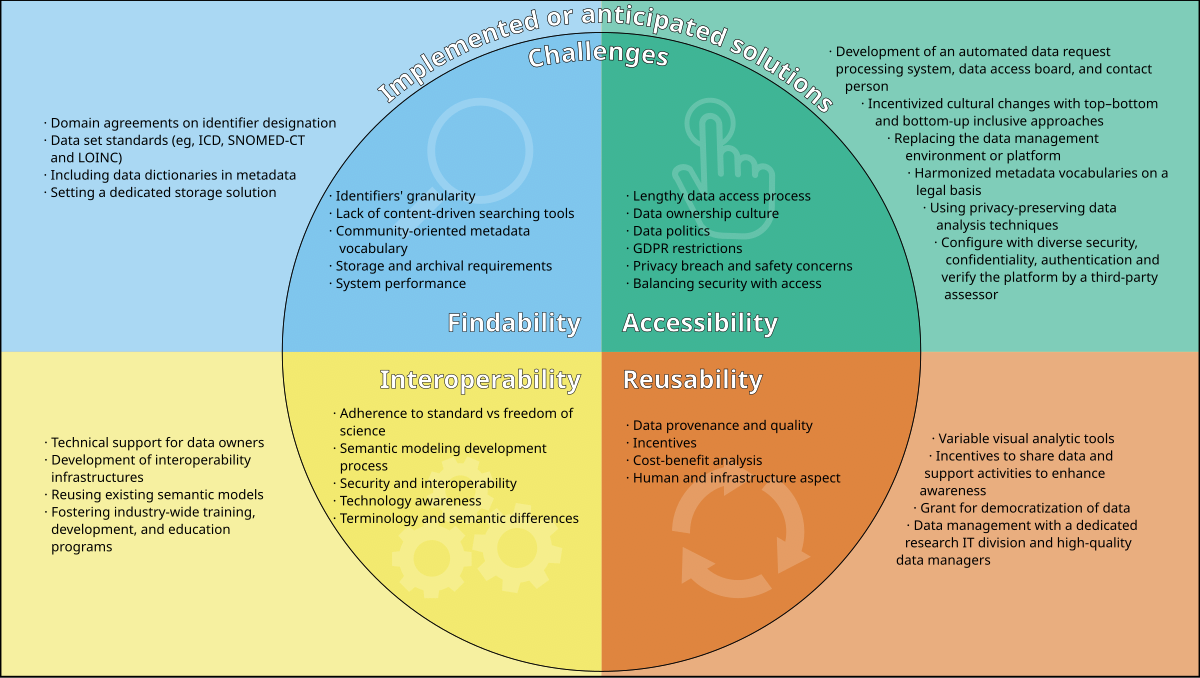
Summary of the challenges and mitigation strategies used in the implementation of the findable, accessible, interoperable, and reusable data principles in health data stewardship. GDPR: General Data Protection Regulation; ICD: International Classification of Diseases; IT: information technology; LOINC: Logical Observation Identifiers Names and Codes; SNOMED-CT: Systematized Nomenclature of Medicine–Clinical Terms.
